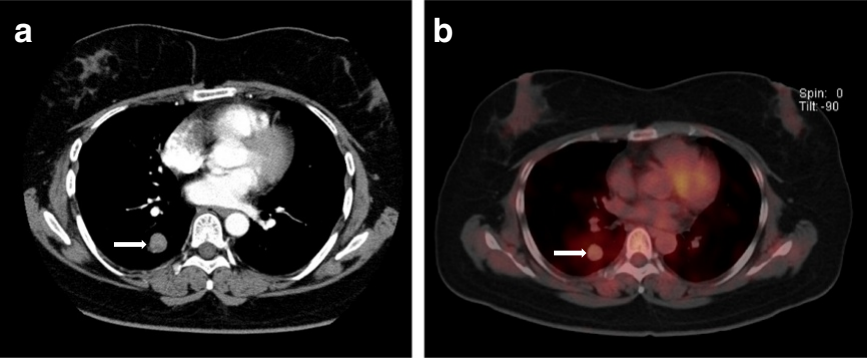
Preoperative computed tomography and positron emission tomography with fluorodeoxyglucose. Axial section of computed tomography (a) and positron emission tomography/computed tomography with fluorodeoxyglucose (b) show slightly high uptake by the right lung mass (arrow).
